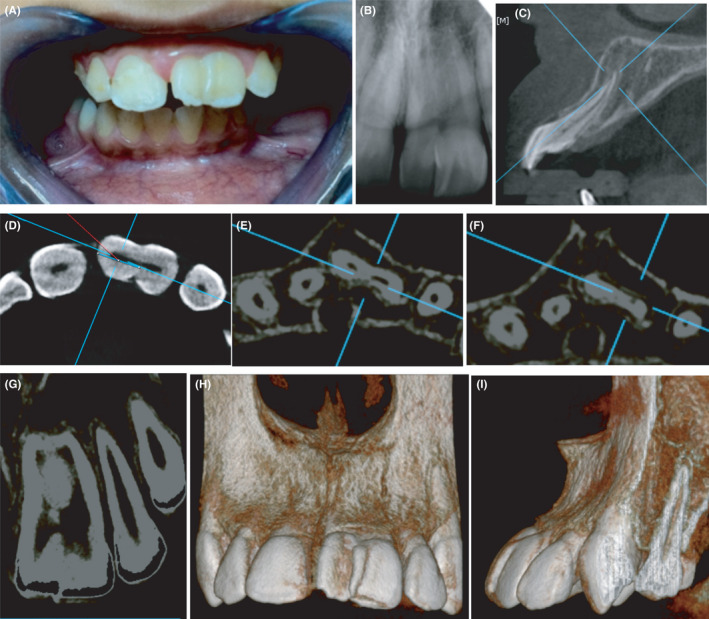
Pre‐operative assessment of the case showing a 12‐yr‐old male patient presenting with malocclusion classified as class II division I and requiring orthodontic treatment. Macrodontia of the left maxillary central incisor is evident. Patient was systemically healthy and his chief complaint was poor esthetics. (A) Pre‐operative frontal view showing a maxillary left “double‐tooth” where the crown of the maxillary left central incisor is fused with a supernumerary tooth; (B) Digital periapical radiograph of the tooth; (C) CBCT sagittal slice showing severe labial proclination of the fused tooth; (D‐F) Axial CBCT slices in the fused tooth at the cervical, middle, and apical thirds of the tooth, respectively. Note the labial opening of the apical foramen; (G) CBCT coronal slice showing the fused tooth with a common root canal system in the coronal up to the middle third followed by separation and rejoining just before the apex; (H) 3D CBCT rendering labial view; (I) 3D CBCT rendering proximal view. To estimate the bone graft amount: the volume of an anticipated cylinder was calculated using the formula : V = hπr^2^ H = 22.83 mm. , R = 3.15 mm. ,V = 22.83 x 3.15x 3.15 x 3.14 = 711. 30 mm^3^ = 0.71130 cubic cm^3^ = 0.7 grams; therefore, approximately 1 gm of graft was calculated to fill the socket
